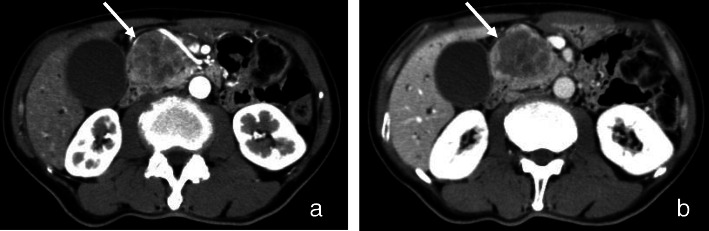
Contrast-enhanced CT revealed a slight enhancement with a poor enhanced spot in the solid tumor with an indistinct border (arrow). a Early phase, b late phase
